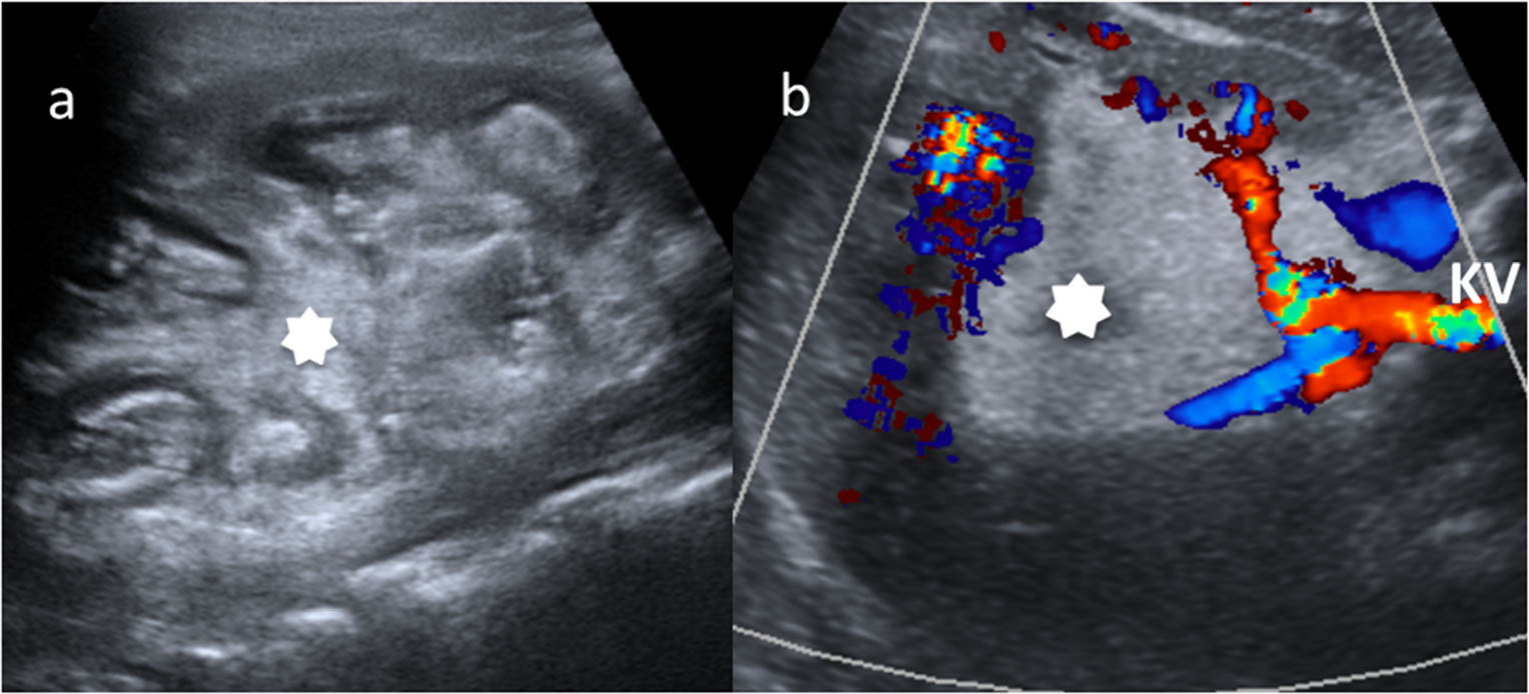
Diffuse XPN in left kidney. a Longitudinal ultrasound shows important inflammatory exudate and fatty proliferation (*white asterisk*) inside the kidney. b Transverse Doppler ultrasound shows kidney vessels (*KV*) passing through the inflammatory and fatty proliferation (*white asterisk*)
